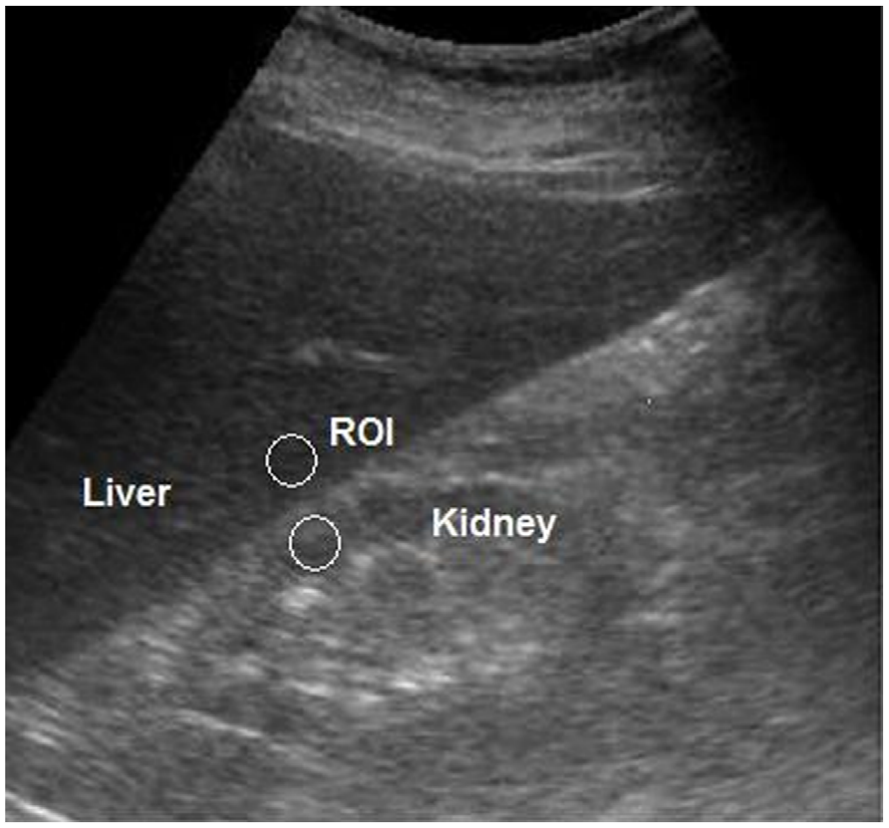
Ultrasound image shows selected regions of interest (ROI) (*circles*).
